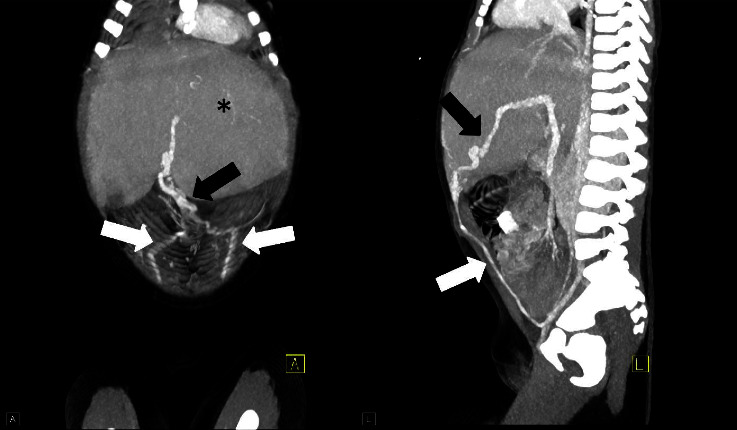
Coronal and sagittal maximum intensity projection CT with IV contrast images shows hepatomegaly with enlarged left lobe (asterisk) and recanalized umbilical vein extending from the left portal vein anteriorly near the umbilicus (black arrow) and joining the bilateral inferior epigastric veins (white arrows) to reach the iliac veins.
